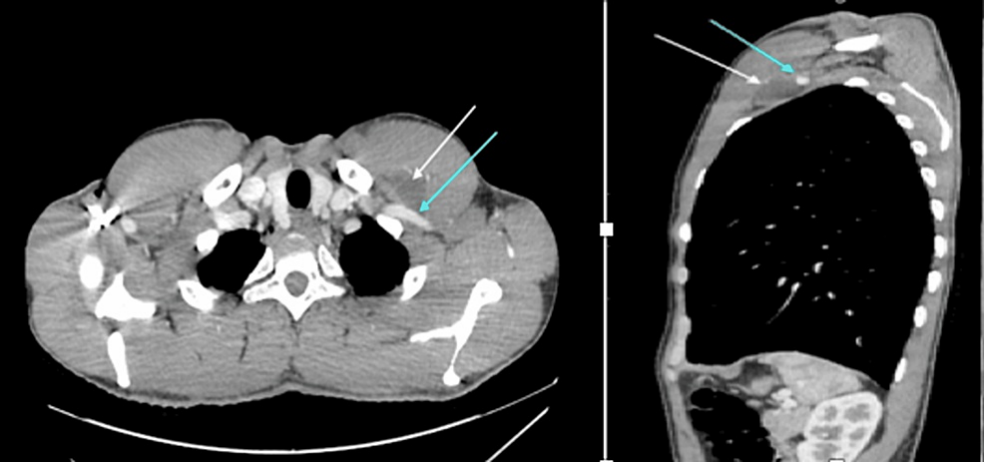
Chest CT scan showed deep cystic formation of the anterior and upper left chest wall. The lesion is anterior and inferior to the left axillary artery and in contact with the left first rib.
